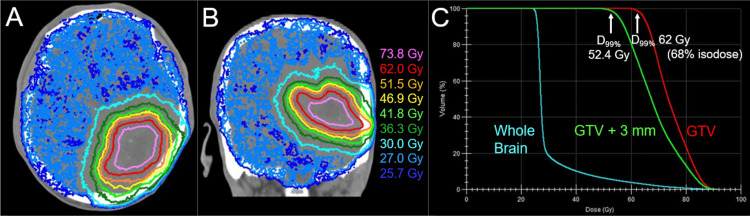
Another alternative plan with dose escalation to the gross tumor for 15-fr radiosurgery combined with simultaneously integrated reduced-dose whole-brain irradiation The images show (A, B) the dose distributions superimposed onto CT images, (A) an axial image, (B) a coronal image and (C) DVHs. (A-C) In the alternative plan, 62 Gy/15 fr is assigned to the D_99%_ of the GTV. The BED_10_ of 62 Gy/15 fr is 87.6 Gy, almost equivalent to a single dose of 25 Gy (BED_10_ 87.5 Gy). The cumulative BED_10_s of the GTV D_99%_ in the original and alternative plans are 102.6 Gy and 87.6 Gy (14.6% reduction), while the total treatment duration is reduced by approximately one-third with the alternative plan. The cumulative BED_2_s of the GTV D_99%_ in the original and alternative plans are 195.2 Gy and 190.1 Gy (2.6% reduction), respectively. The cumulative BED_2_s of the GTV + 3 mm D_99%_ in the original and alternative plans are 157.1 Gy and 143.9 Gy (8.4% reduction), respectively. D_99%_: a minimum dose encompassing at least 99% of the target volume; GTV: gross tumor volume; GTV + 3 mm: GTV plus a uniform 3-mm margin; CT: computed tomography; DVH: dose-volume histogram; fr: fraction(s); BED_10/2_: a biologically effective dose based on the linear-quadratic formula with an alpha/beta ratio of 10/2
